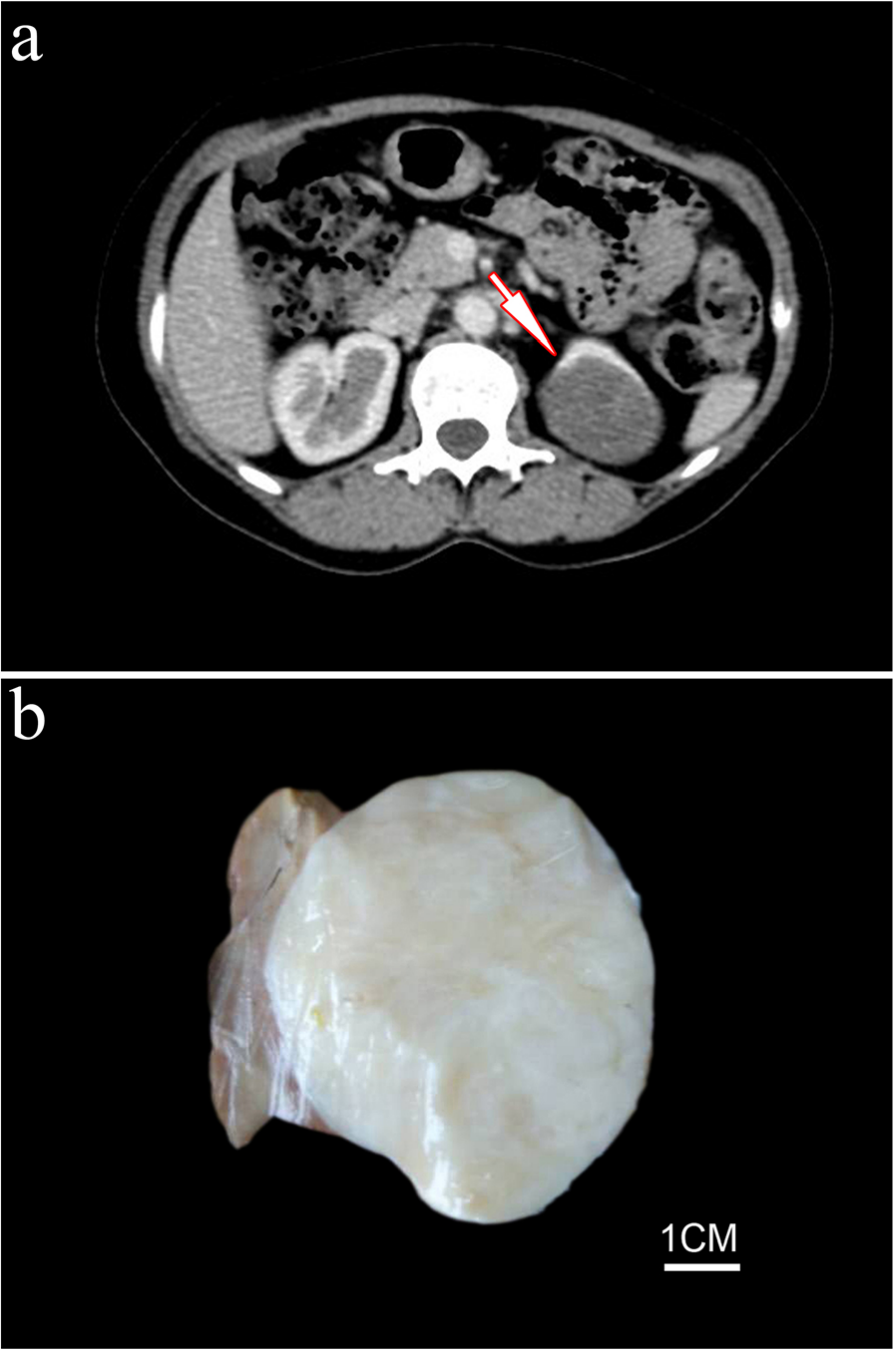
Case 1. CT scan showed a sharply defined mass involving the upper pole of the left kidney (A, *as indicated by arrow*). Gross examination revealed a well-demarcated, round and whitish, solid tumor (B)
